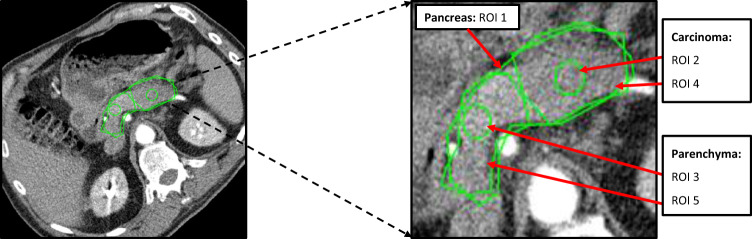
CT perfusion image with marked regions of interest (ROIs). CT image showing ROIs used for evaluation of a patient with pancreatic adenocarcinoma. Polygonal ROI1 outlines the whole pancreatic region. The carcinoma region has been marked by polygonal ROI4 and inside it, a small circular ROI2 has been marked to include carcinoma tissue only, excluding part of any other tissue or blood vessels. Similarly, the parenchyma region has been marked by a polygonal ROI5 and inside it, a small circular ROI3 has been placed to include parenchyma tissue only, excluding any other tissue or blood vessels.
